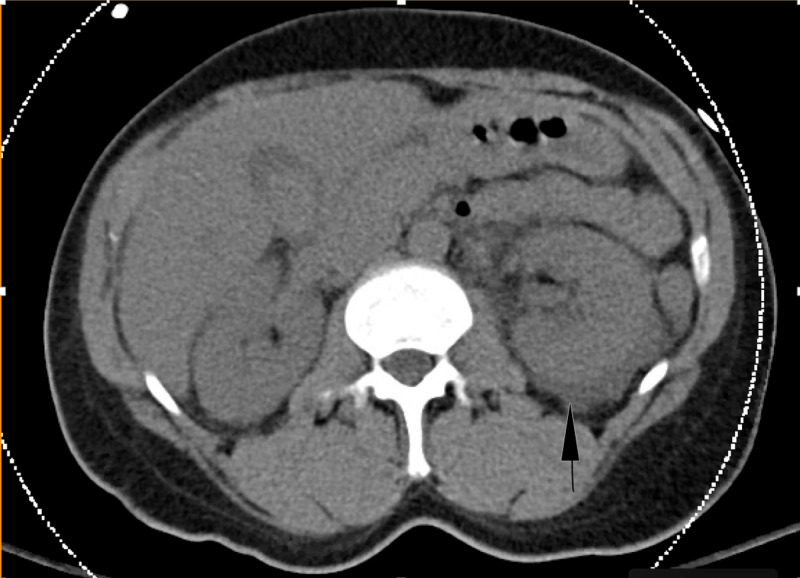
CT abdomen and pelvis without contrast: showing fluid surrounding both the kidneys with moderate amounts of fluid in the pelvis. Gall bladder showed a filling defect, thought to be secondary to sludge/calculus.
